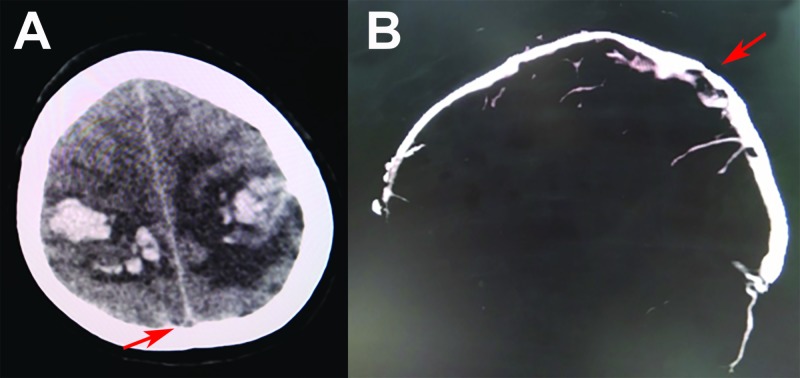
Initial radiographic evidence of cerebral venous thrombosis (CVT) A) Initial brain computed tomography (CT) showing bilateral hemorrhagic infarctions with a non-specific distribution; the empty delta sign is observed (arrow); B) CT venography shows evidence of thrombus in the posterior superior sagittal sinus (arrow).
